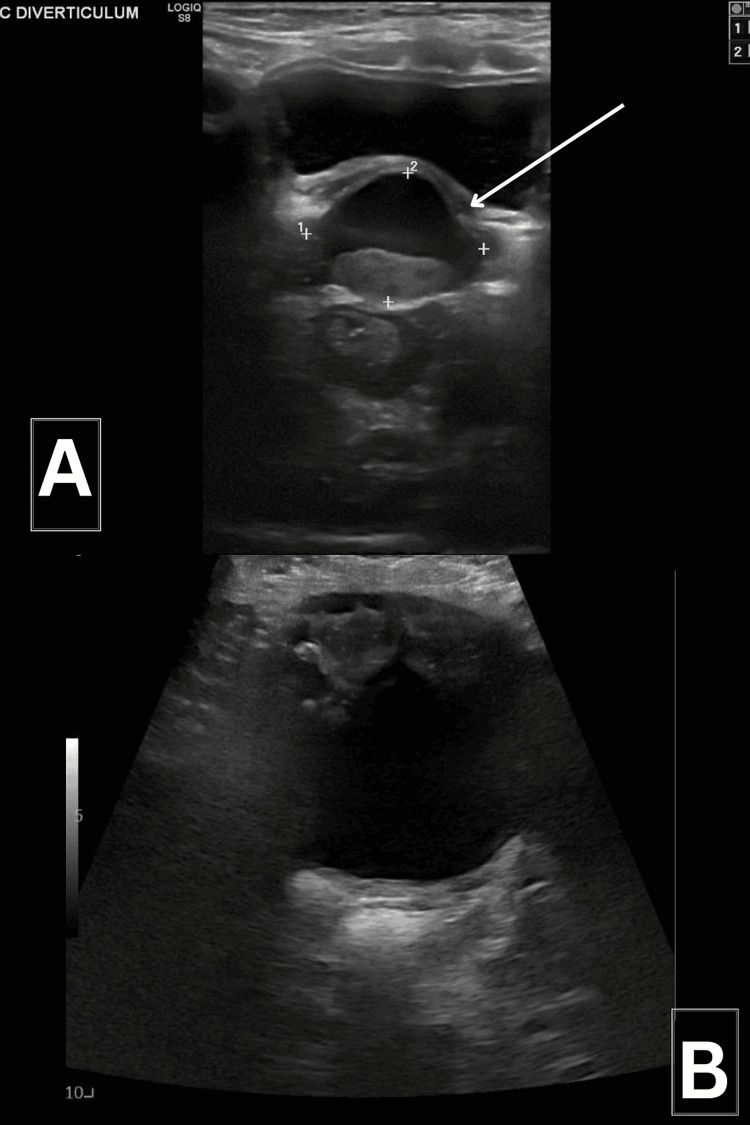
Bladder ultrasound (A) prior to surgical excision (B) postoperatively after the successful laparoscopic excision of the prostatic utricle cyst
